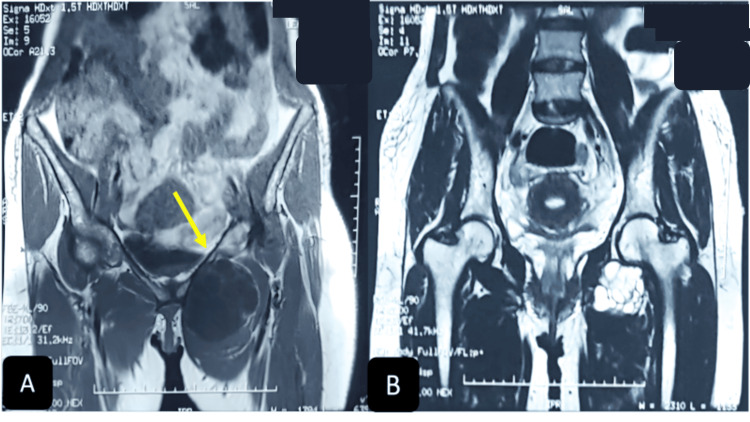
Coronal MRI section depicting a left inguinal mass involving the pectineus muscle, exhibiting hypo T1 signal (A) and hyper T2 signal (B), containing thin-walled vesicles that do not enhance after injection.
